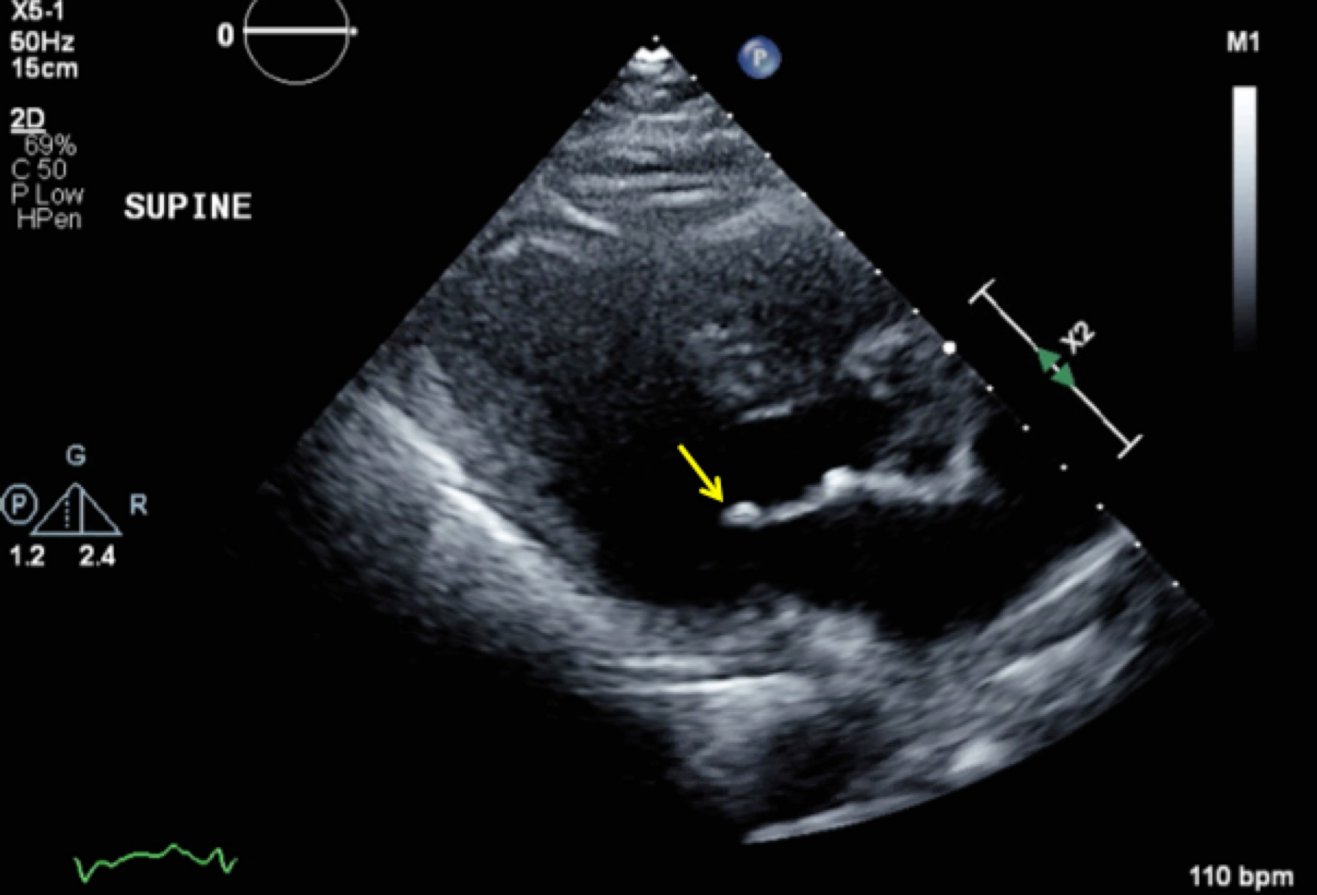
Parasternal long-axis view of the transthoracic echocardiogram (TTE) showing a 0.5 cm vegetation in the anterior leaflet of the mitral valve (yellow arrow)
